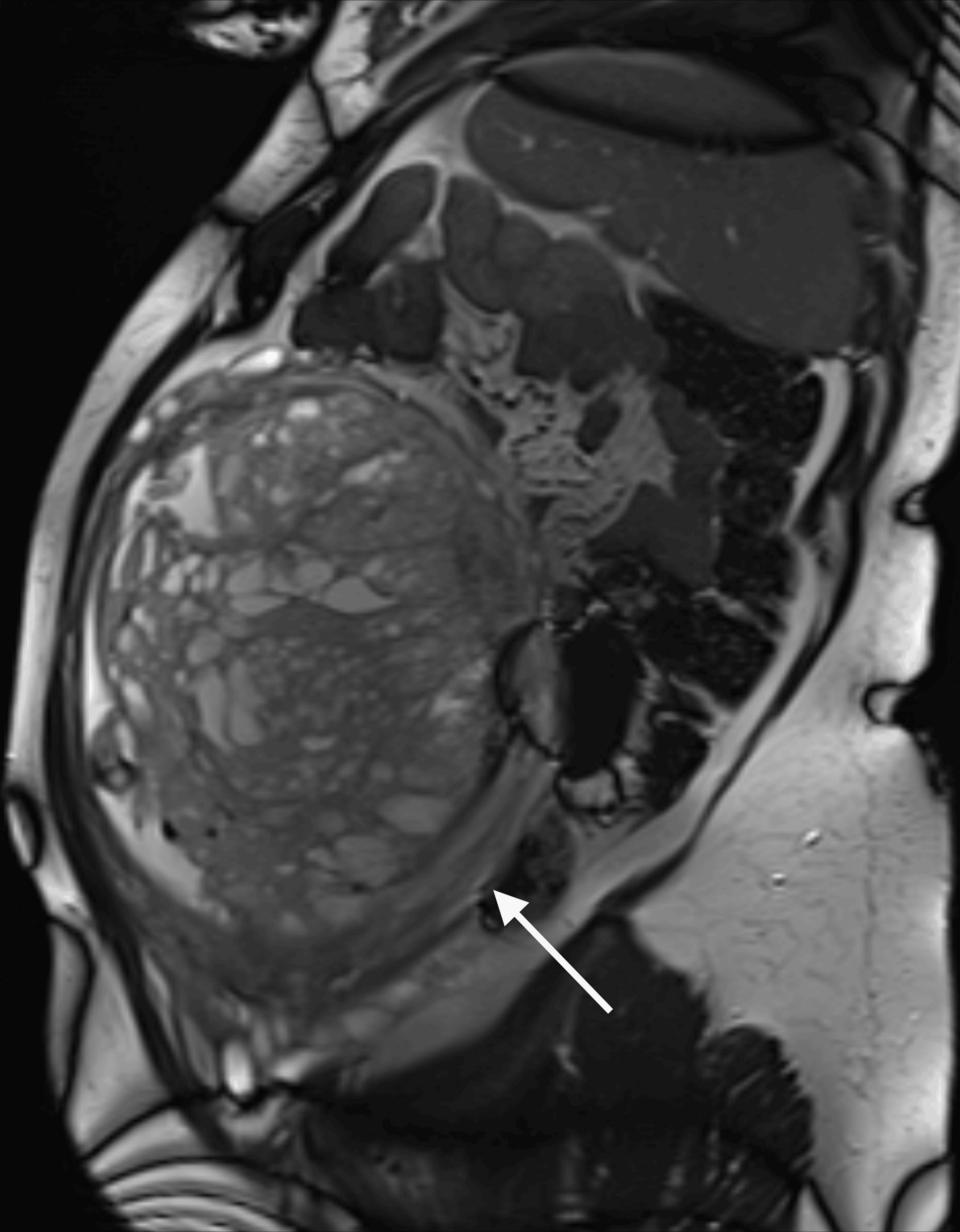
MRI at 28+0 weeks confirming placentomegaly with cystic lesions associated with placental mesenchymal dysplasia The arrow points to the cystic placenta seen on the MRI
